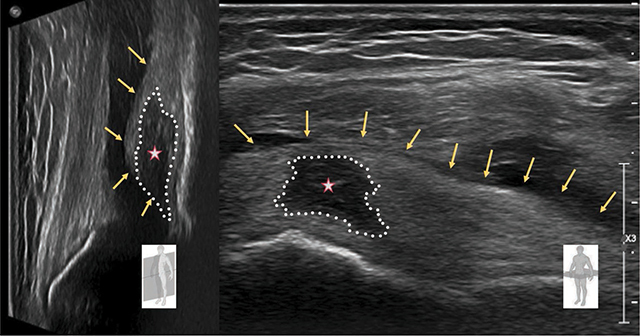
Ultrasonography of the knee. Joint effusion and hypoechoic edema with enlargement of the prefemoral fat pad (asterisk).
